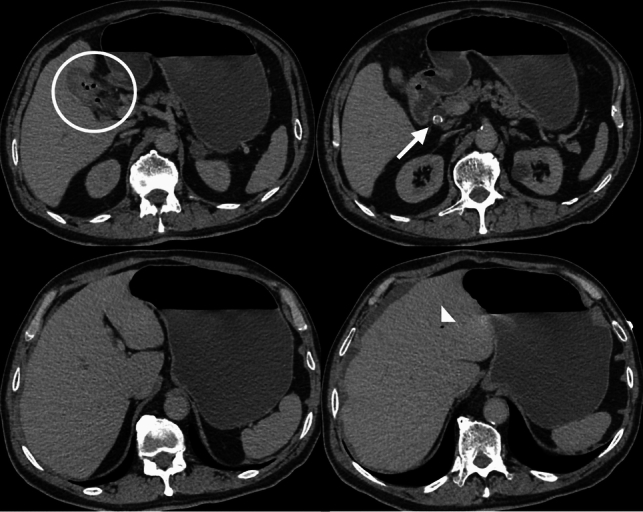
IV contrast-enhanced axial CT images of a 63-year-old female patient with Bouveret’s syndrome. A bilio-duodenal fistula with gallbladder wall thickening and air densities is noted (circle). A large gallstone is impacted in the duodenal bulb (arrow). Gastric dilatation and pneumobilia are evident, consistent with Rigler’s triad
